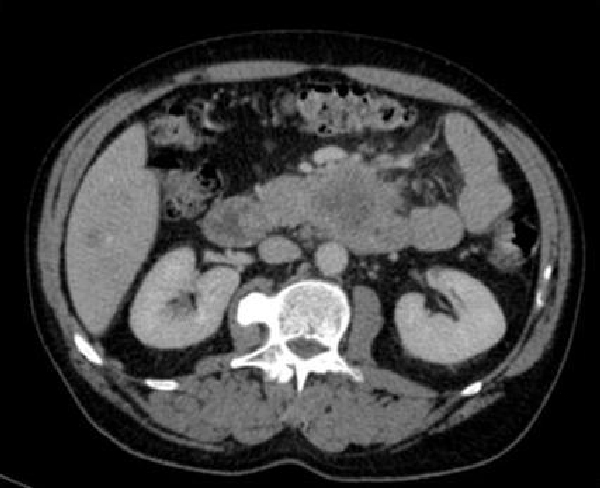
CT scan demonstrated a mass, arising from the uncinate process of the pancreas.
